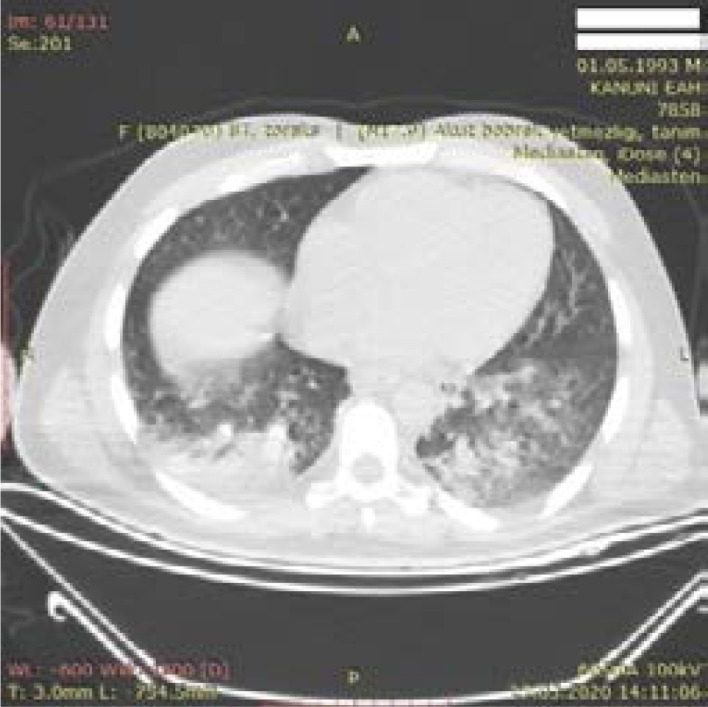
Typical medial CT finding - 27-year-old female patient – Ground glass densities in consolidated form in lower lobes of both lungs
